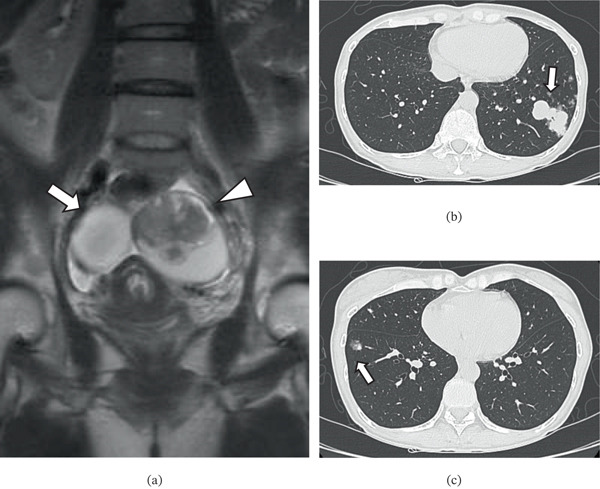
(a) Preoperative magnetic resonance imaging of both ovaries. T2‐weighted imaging shows bilateral ovarian masses with cystic and solid components (arrow: right ovary; arrowhead: left ovary). (b, c) Computed tomography images of the lungs showing multiple nodules (arrows) 1 year after the patient underwent hysterectomy and salpingo‐oophorectomy.
